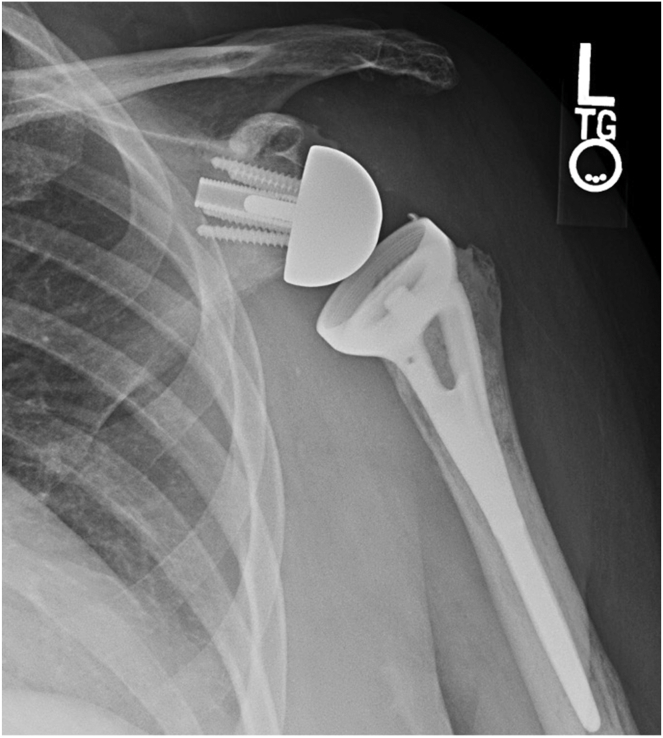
Post-operative true anteroposterior radiograph of the shoulder after rTSA with an allograft reconstruction of a central glenoid defect. *rTSA*, reverse total shoulder arthroplasty.
